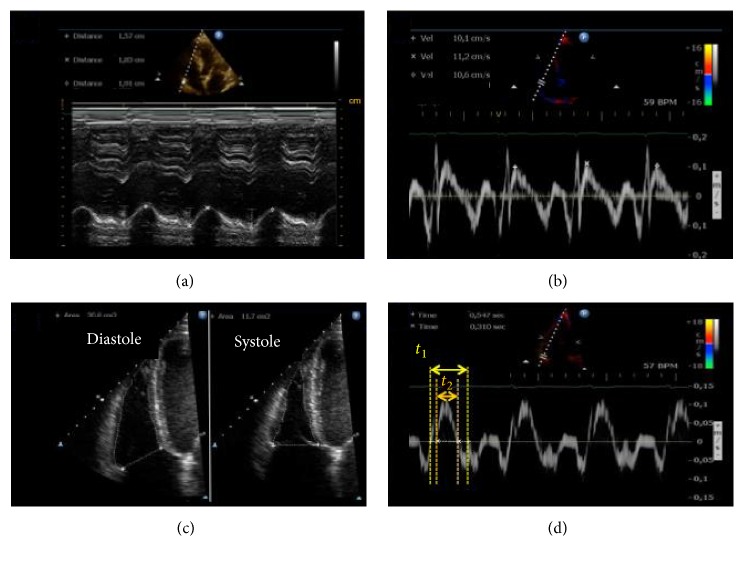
Echocardiographic conventional parameters of right ventricle (RV) evaluation. (a) Tricuspid Annular Peak Systolic Excursion (TAPSE) from M-mode. (b) Systolic wave velocity (*S*′) of the lateral portion of tricuspid annulus; (c) area measurements in diastole and systole from bidimensional images to calculate fractional area change (FAC); (d) time measurements from tissue Doppler curves in tricuspid annulus to calculate myocardial performance index (MPI). [13]. Adapted with permission from Elsevier, license number 4143761084119.
